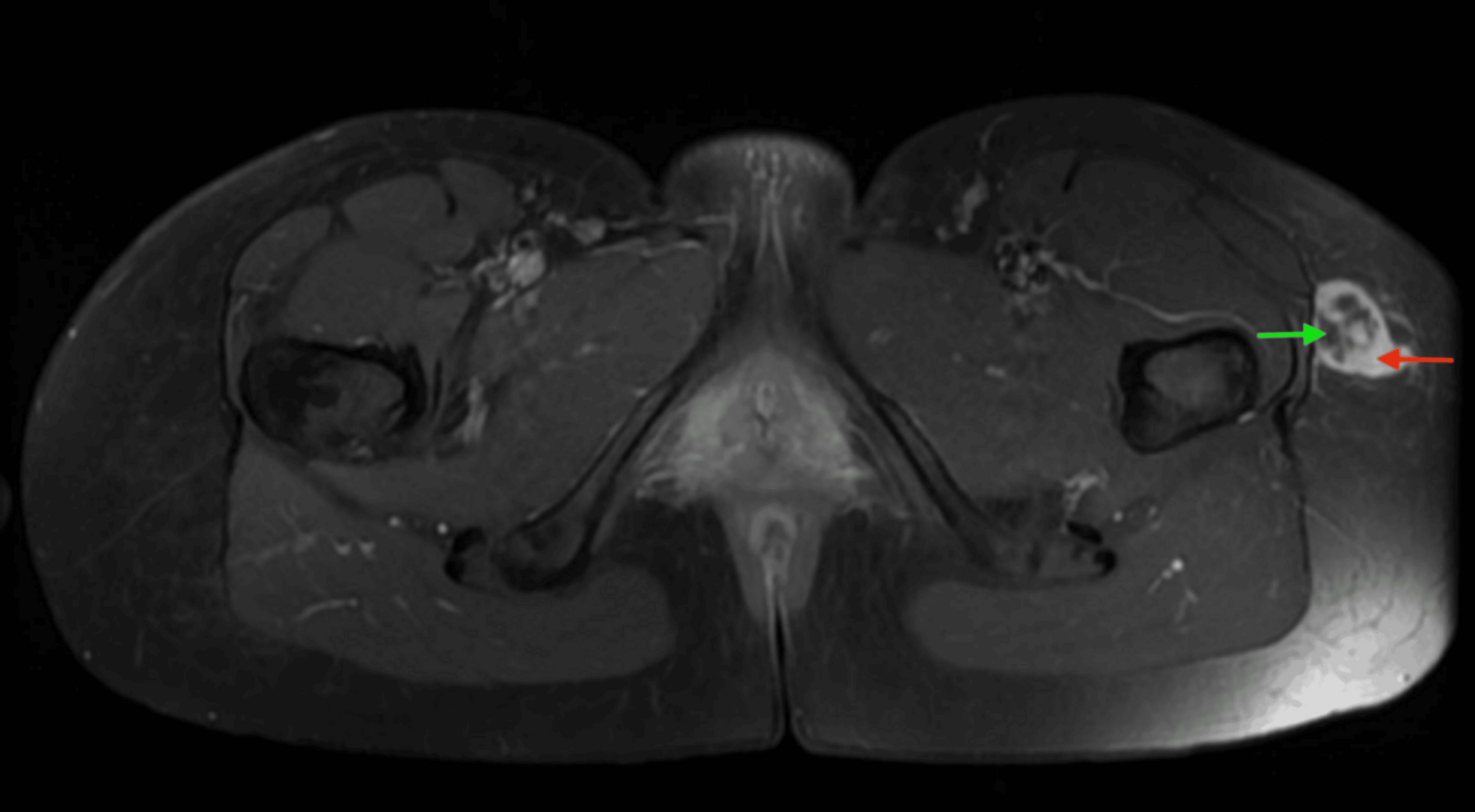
Axial contrast-enhanced T1-weighted fat-suppressed pelvic MRI shows a well-circumscribed superficial nodule along the lateral aspect of the left hip, adjacent to the tensor fasciae latae (red arrow), with heterogeneous peripheral enhancement and a central non-enhancing area, consistent with a hypocellular or myxoid stromal component (green arrow). No deep fascial breach, bone involvement, or neurovascular encasement is present.
